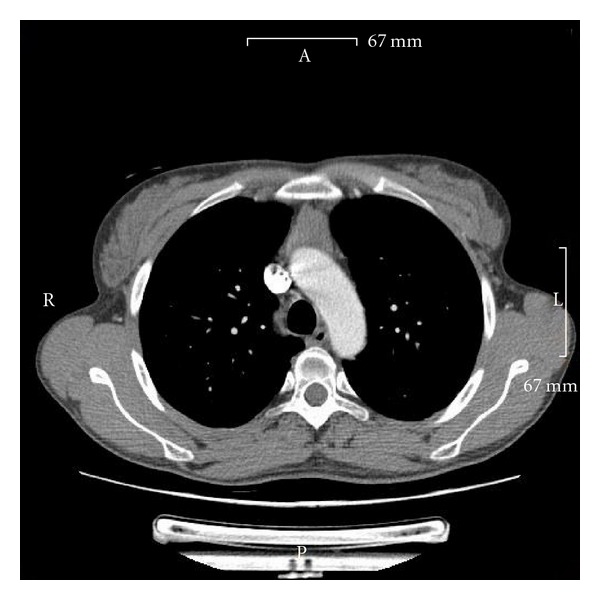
CT scan of the thorax showing the enlarged mediastinal mass in front of the aortic arch.
